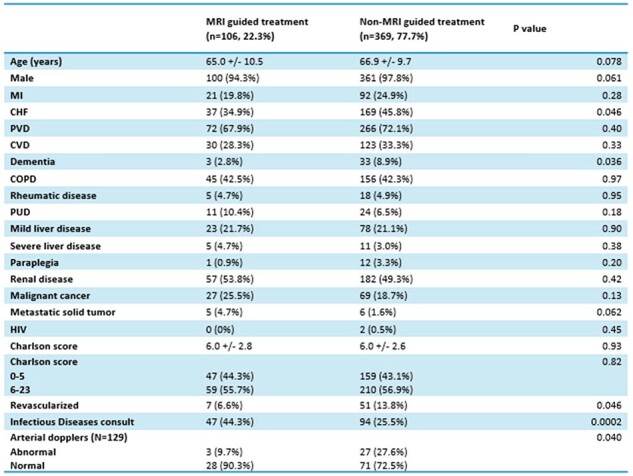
Patient characteristics and comorbidities MRI=magnetic resonance imaging, MI=myocardial infarction, CHF=congestive heart failure, PVD=peripheral vascular disease, CVD=cardiovascular disease, DM=diabetes mellitus, COPD=chronic obstructive pulmonary disease, PUD=peptic ulcer disease, HIV=human immunodeficiency virus
